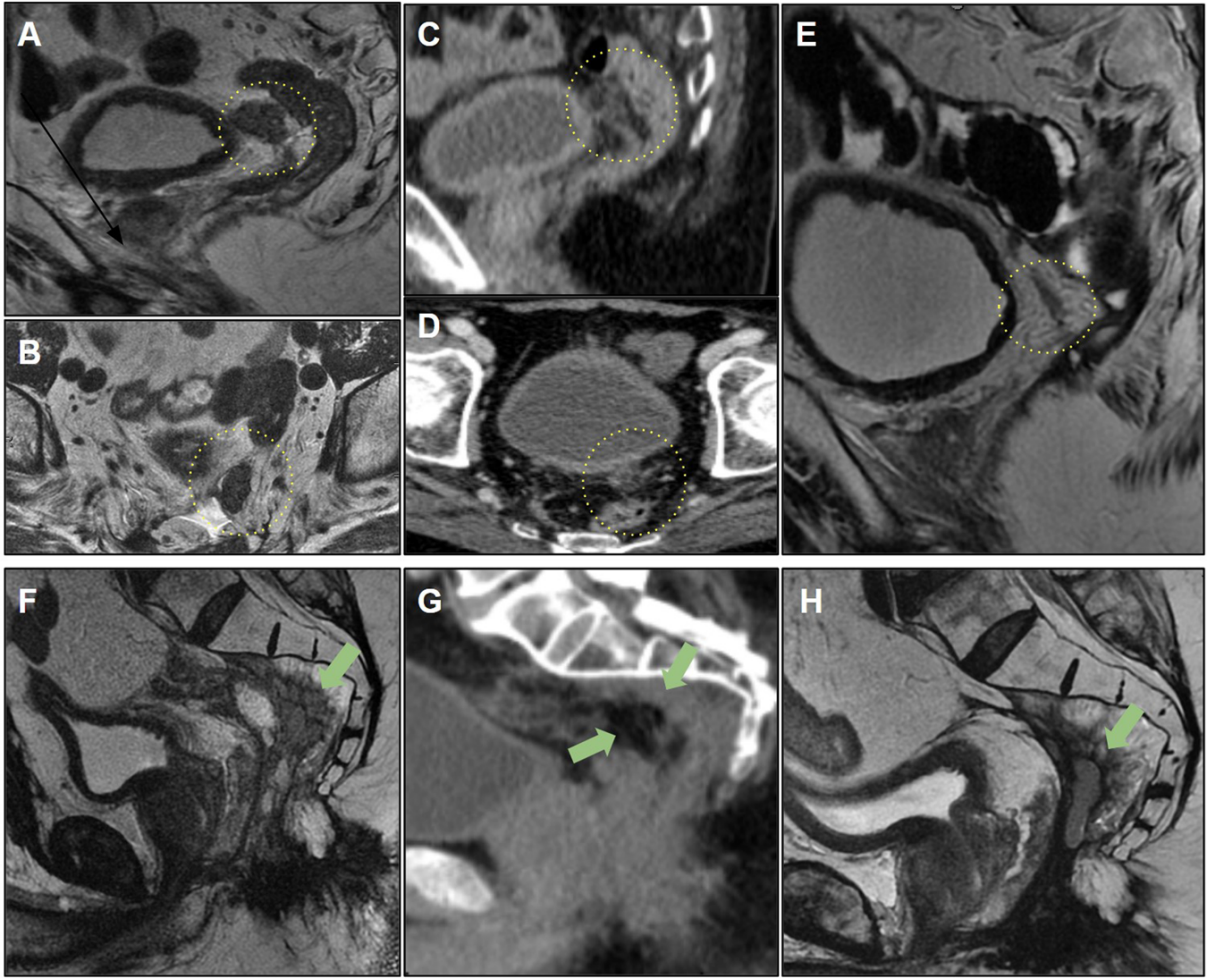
A, B The patient underwent rectosigmoidectomy (pT2N0). MRI 9 months after surgery revealed a nodular lesion with intermediate T2 signal anterior to the mesocolon (yellow circle). Suspected tumor recurrence. C, D Computed tomography (CT) demonstrating a fatty nodular lesion consistent with steatonecrosis (yellow circle). E Steatonecrosis focus reduced after 4 years of follow-up, and the absence of pelvic recurrence was observed. F Another case of a patient who underwent abdominoperineal amputation with omental flaps. Solid tissue on the surgical resection bed was misinterpreted as recurrence (green arrow). G Comparison of CT scans shows omental flaps mimicking pelvic recurrence (green arrow). H Follow-up examinations reveal tissue shrinkage with increased fibrotic content. Stability was observed for 5 years, supporting no signs of recurrence and indicating a pattern of postsurgical changes (green arrow)
